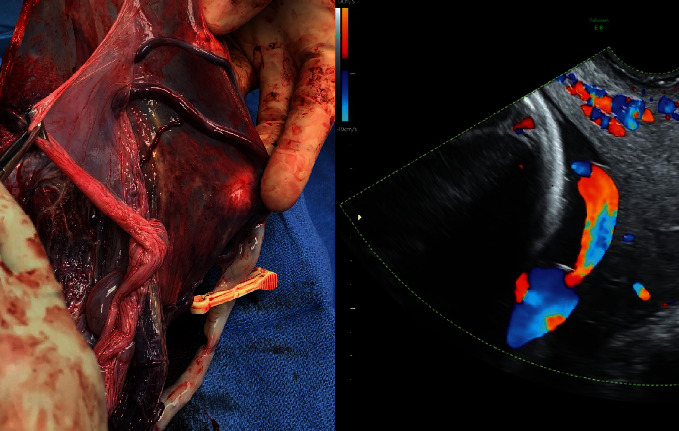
Gross image of the placenta with corresponding ultrasound. Ultrasound image shows proximity of fetal vessel to cervix.
